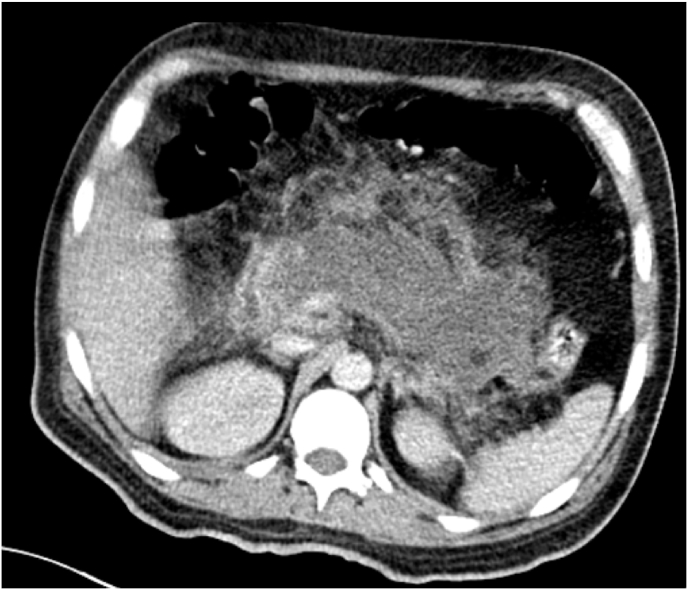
abdominal CT scan revealing a pancreas increased in size, losing its physiological lobulations, heterogeneously enlarged after injection of contrast, containing some hypodense areas of necrosis, associated with significant infiltration of the surrounding fat with multiple peri-pancreatic necrosis flows, opposite the anterior para-renal fascia, bilaterally peri-splenic at the level of the parietal colic gullet and fusing at the mesenteric level.
